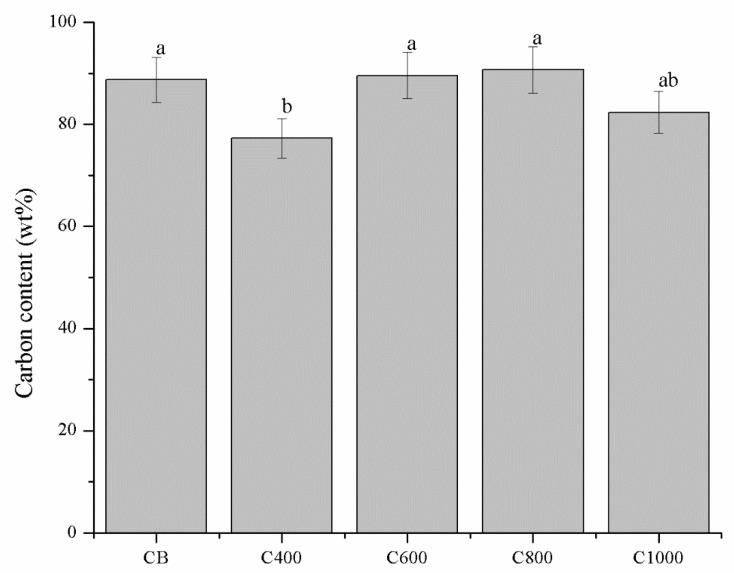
Carbon content of biochars and CB. Evaluated though energy dispersive X-ray (EDX) analysis. Error values were reported as 5% of the detected values according with Laskin et al. [65]. Columns marked with different letters are significantly different (*p* < 0.05).
